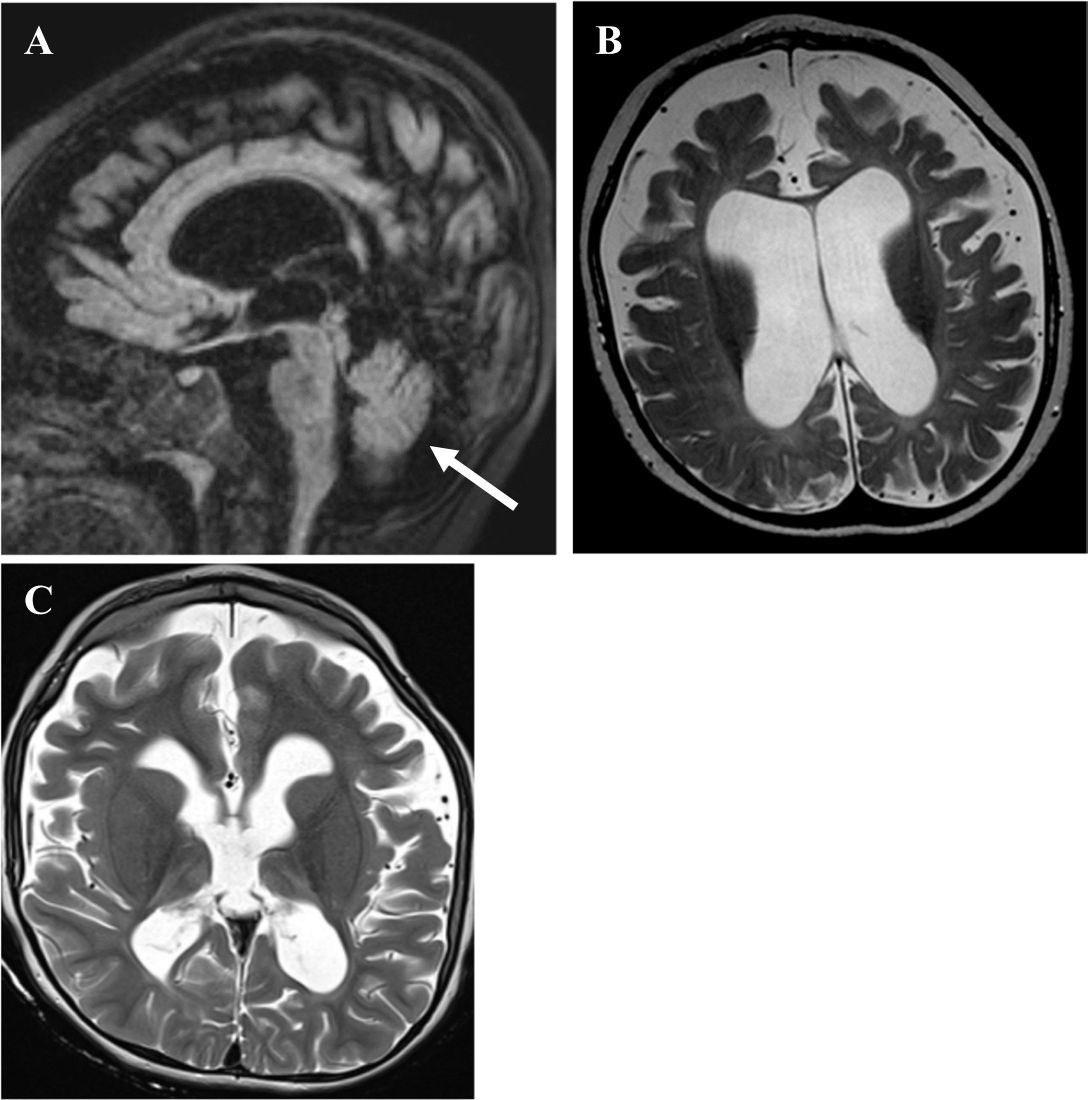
Brain MRIs of a patient with *RARS2*-related Mitochondrial Disorder. (Top Left) Sagittal FLAIR image of brain MRI at twelve months of age showing large subarachnoid spaces, ventriculomegaly, and generalized brain atrophy. There is a minimal reduction in brainstem size, and the volume of the cerebellum is preserved (white arrow). (Top Right) Axial T2W image at twelve months of age demonstrating *ex vacuo* enlargement of the ventricles and widening of the subarachnoid spaces. (Bottom Left) Axial T2W image at four years and six months of age demonstrating new T2 hyperintense signals in the bilateral thalami in addition to generalized volume loss, thinning of the corpus callosum, *ex vacuo* enlargement of the ventricles, and widening of the subarachnoid spaces
